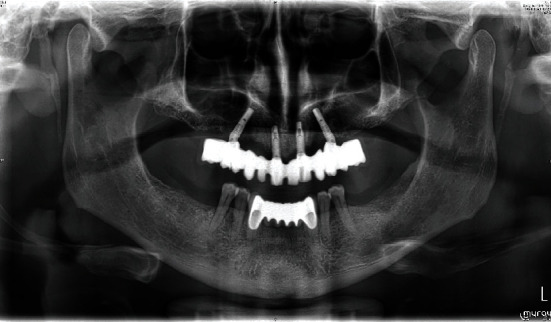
Orthopantomogram to control the definitive FDP.
